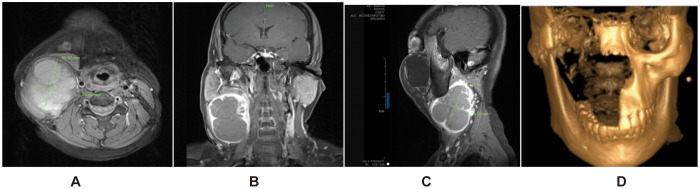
The disease of the patient as depicted on the MRI and CT scans. (A). Axial MRI, (B). Coronal MRI, (C). Sagittal MRI, (D). CBCT reconstruction.
